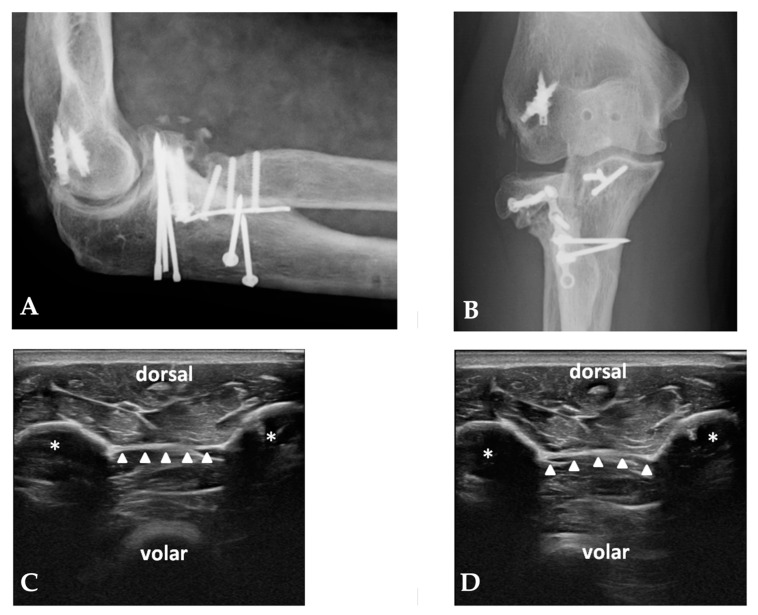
A Monteggia-like lesion in a 60-year-old female patient. The last follow-up of the patient after reintervention for contracture release and posterior ulnar plate removal. The X-rays show (A,B) an asymptomatic non-union of the radial head and radial plate breakage. At the last follow-up, a bilateral US examination of the cbIOM was performed. (C) shows a dynamic examination of the non-injured side, while (D) shows a slight increase in laxity on the affected side. The white asterisks represent the forearm bones. The white arrowheads point to the interosseous membrane.
